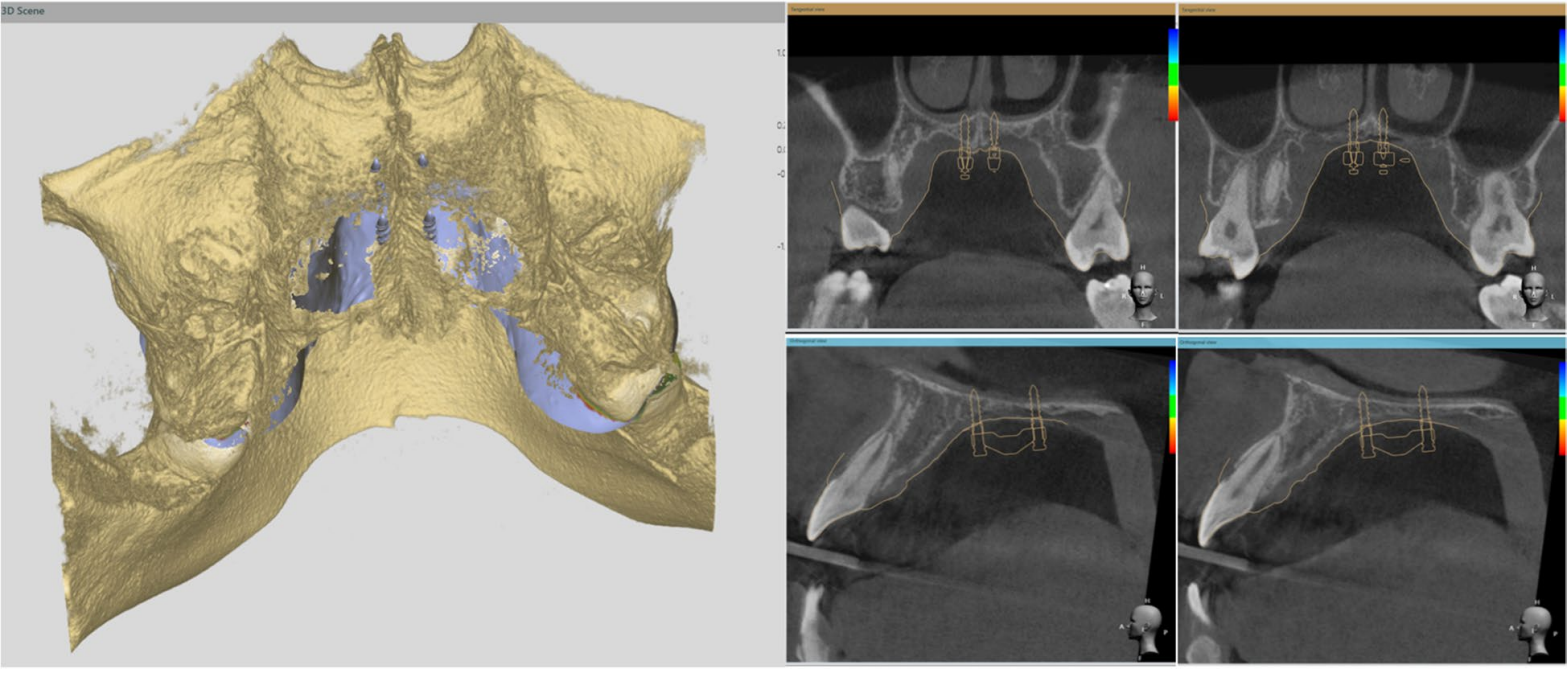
Importing merged surface with CBCT and verification of appliance position and determination of the appropriate length of the mini-screws. The actual distance from the inferior surface of MSE to the superior cortical plate of the maxilla can be measured, which were 9.51 mm (anterior) and 6.97 mm (posterior). Therefore, 11 mm for the anterior and 9 mm for the posterior screws were chosen to achieve bicortical engagement
